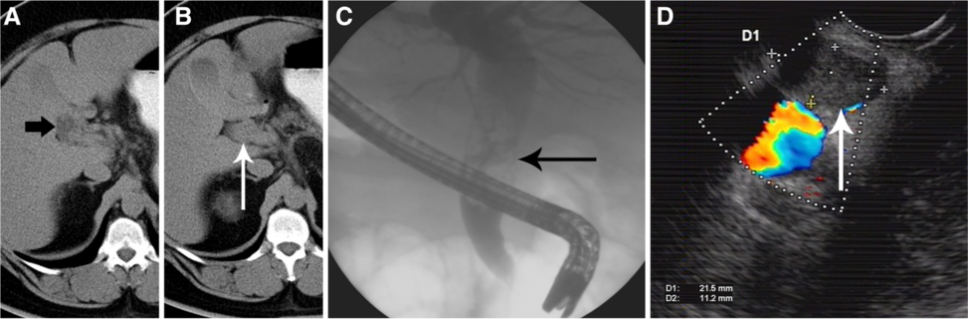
Radiographic findings of the pyloric gland adenoma of the cystic duct. Abdominal computed tomography revealed markedly dilated intrahepatic bile ducts (A, arrow), and a polypoid tumour in the common hepatic duct just below the bifurcation (B, arrow). Endoscopic retrograde cholangiopancreatography confirmed the polypoid mass lesion (C, arrow) and demonstrated consecutive dilation of the central intrahepatic bile ducts. Endosonography verified the polypoid intraluminal tumour of 2.1 x 1.1 cm in the common hepatic duct (D, arrow) next to the portal vein.
